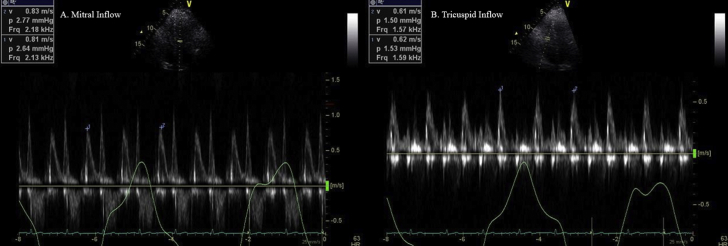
On-treatment Doppler recording of (A) mitral and (B) tricuspid inflow with a respirometer showing no evidence of constrictive physiology.
